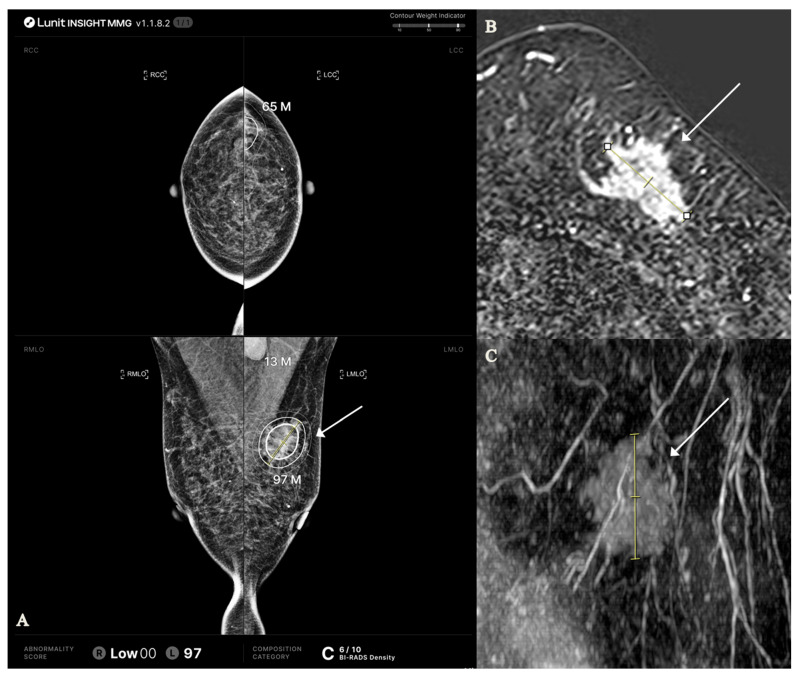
AI-CAD mammography and MRI findings in a 53-year-old woman with invasive ductal carcinoma, grade III (HR+/HER2−, Ki-67 index 85%). (A) AI-CAD mammography shows heterogeneously dense breasts (category C, density score 6/10) and a high abnormality score (97) on the left mediolateral oblique view. The lesion was classified as a mass. Tumor size along inner, middle, and outer contours was 2.6, 3.8, and 4.6 cm, respectively (T2 stage for all). A partially visualized enlarged axillary lymph node was also noted. (B,C) Contrast-enhanced MRI reveals an irregularly enhancing mass in the corresponding area. The lesion measured 2.8 cm on the axial view and 3.8 cm on the maximum intensity projection image; the maximal diameter (3.8 cm) was used for T2 staging. Arrows indicate the lesion; yellow lines represent caliper-based measurements. The outer contour in A was omitted due to visual overlap with other lines. AI-CAD = artificial intelligence-based computer-aided detection; HR = hormone receptor; HER2 = human epidermal growth factor receptor 2; MIP = maximum intensity projection.
